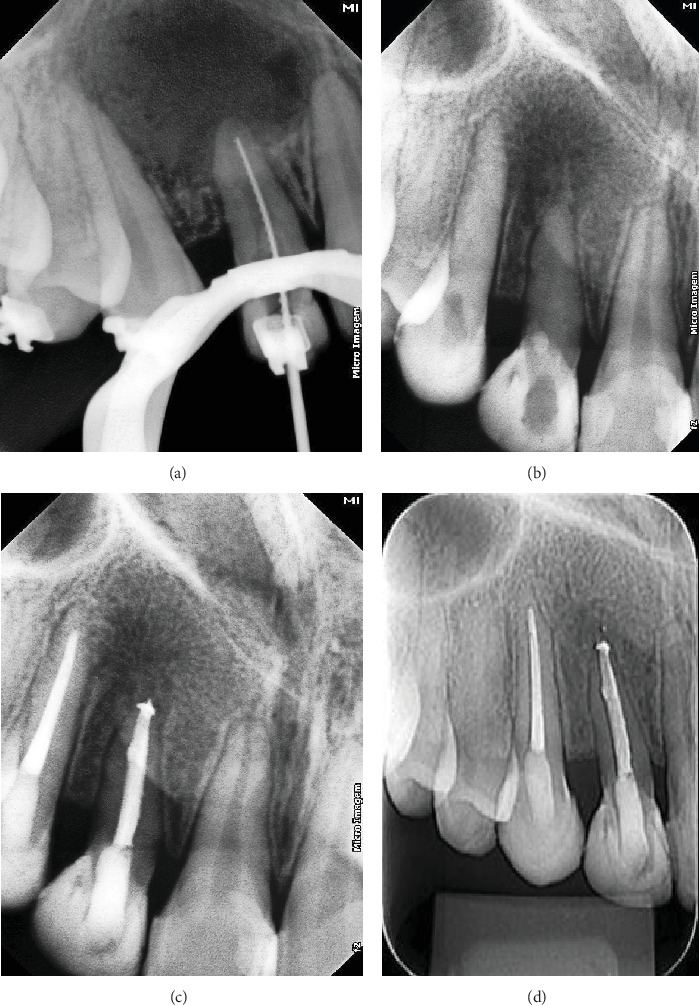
(a) The working length determination radiograph. (b) Application of calcium hydroxide as an intracanal dressing. (c) Final obturation of the root canal. (d) Follow-up visit 7 months later, demonstrating significance in the periapical radiolucency.
